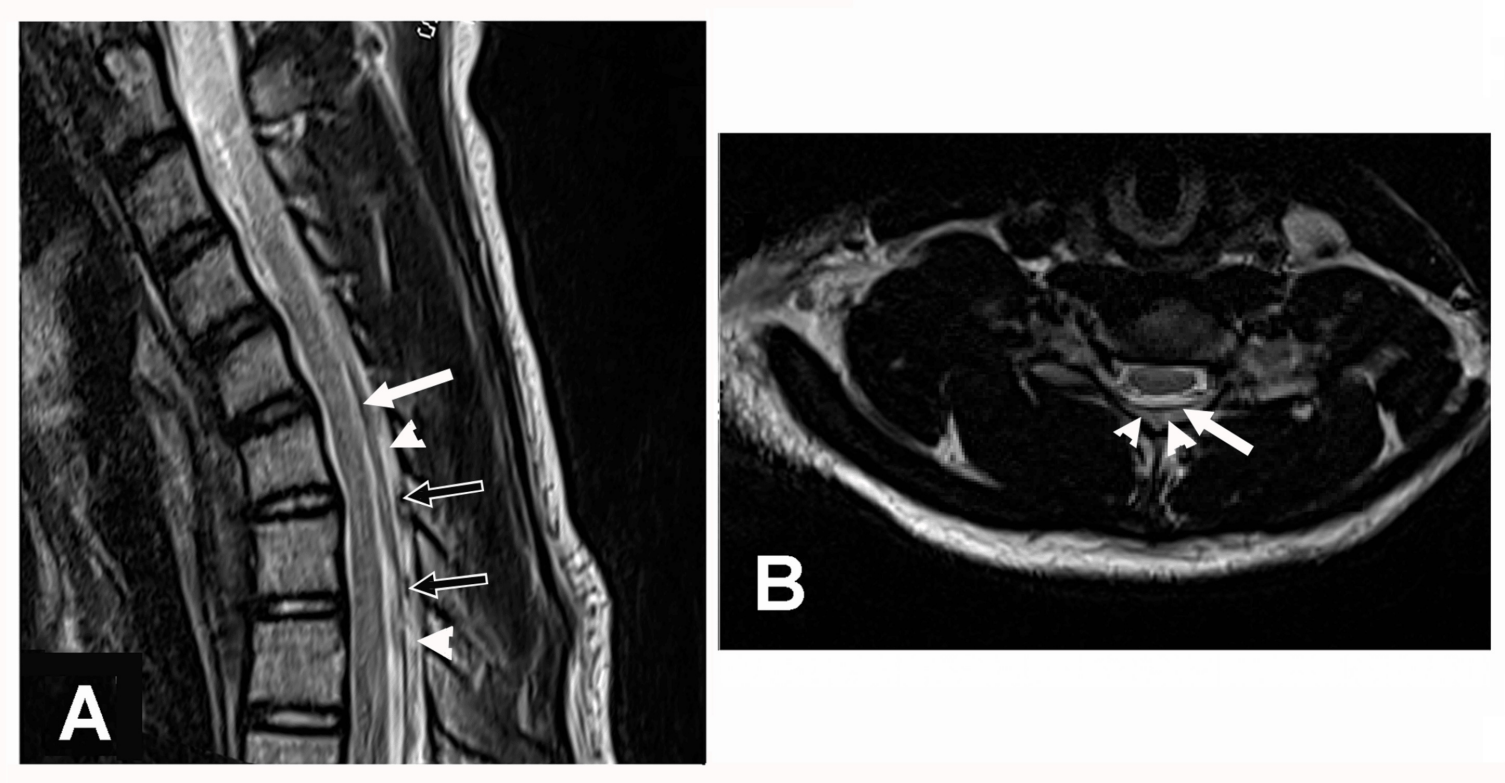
T2-weighted cervical spine MRI scan in the flexed position Case 1: T2-weighted cervical spine MRI scan in the flexed position. (A) Sagittal view demonstrating anterior displacement of the spinal cord, anterior displacement of the posterior dura (arrow), enlarged epidural veins (black arrows), and thickened epidural space (arrowheads). (B) Axial view showing slight atrophy of the left side of the spinal cord and the posterior margin of the thickened epidural space (arrowheads). The arrow signifies posterior dura. MRI: magnetic resonance imaging
